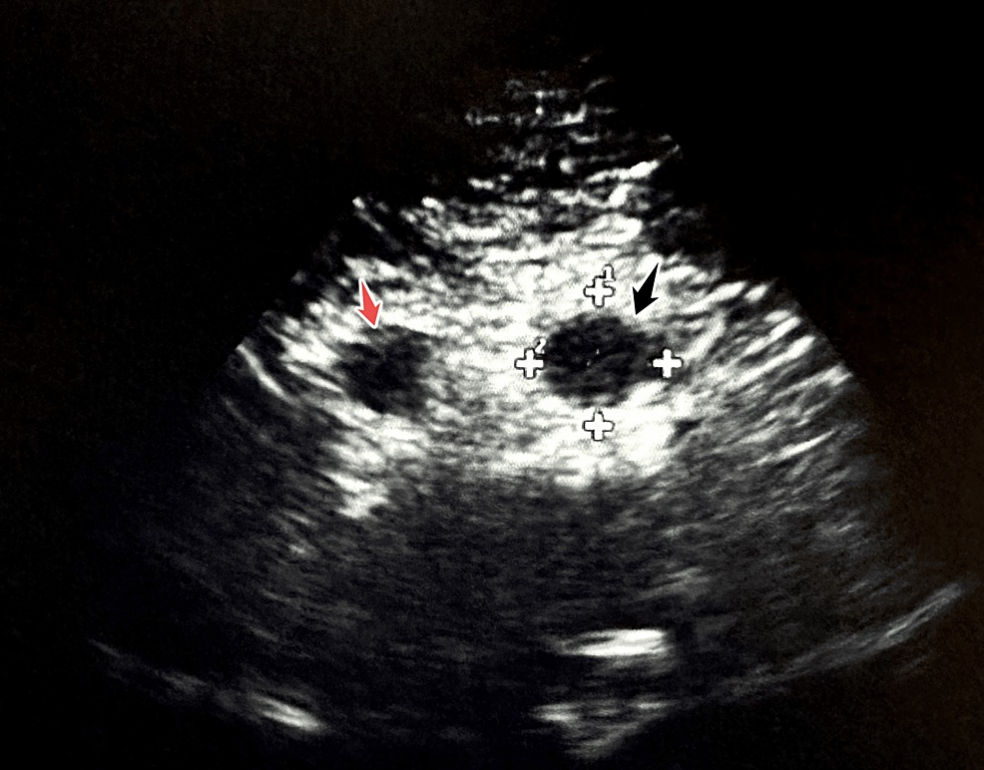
Abdominal ultrasound demonstrating the suprarenal (red arrow) and the infrarenal (black arrow) aneurysm separated by the aorta measuring 2.9 cm.
